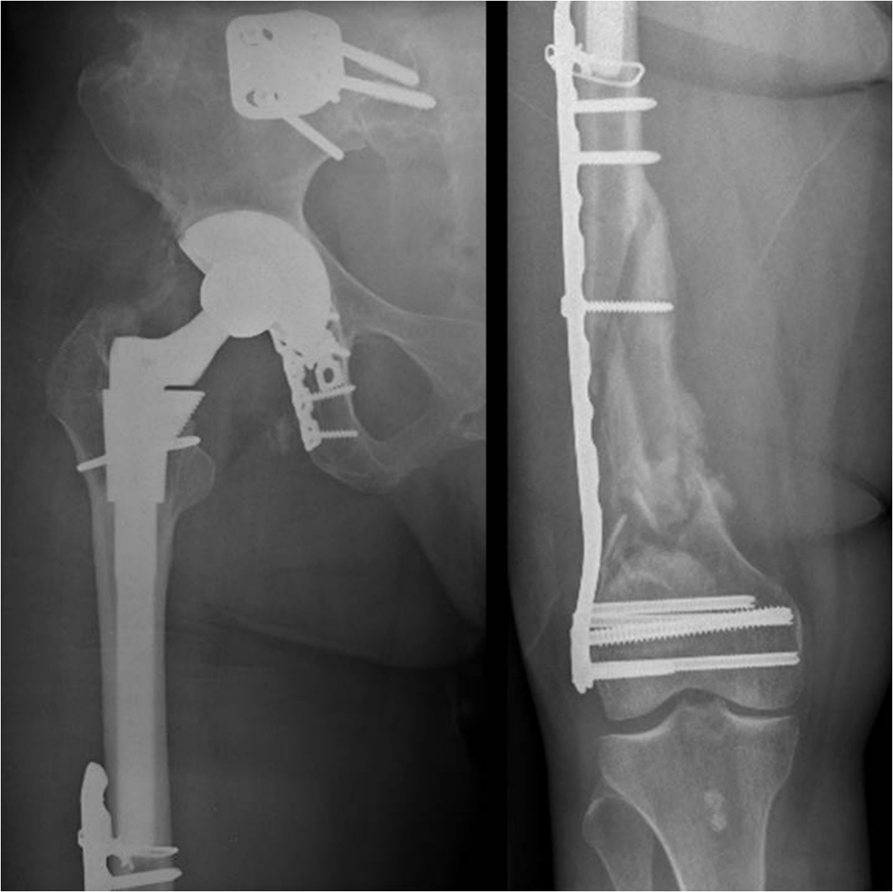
Imaging of the right hip and femur of a 46-year old female patient at first presentation to our institution. Plain radiographs demonstrate a right sacro-iliac joint fusion, right hip arthroplasty after acetabular fracture fixation, and bridge plating of a right distal femur fracture.
